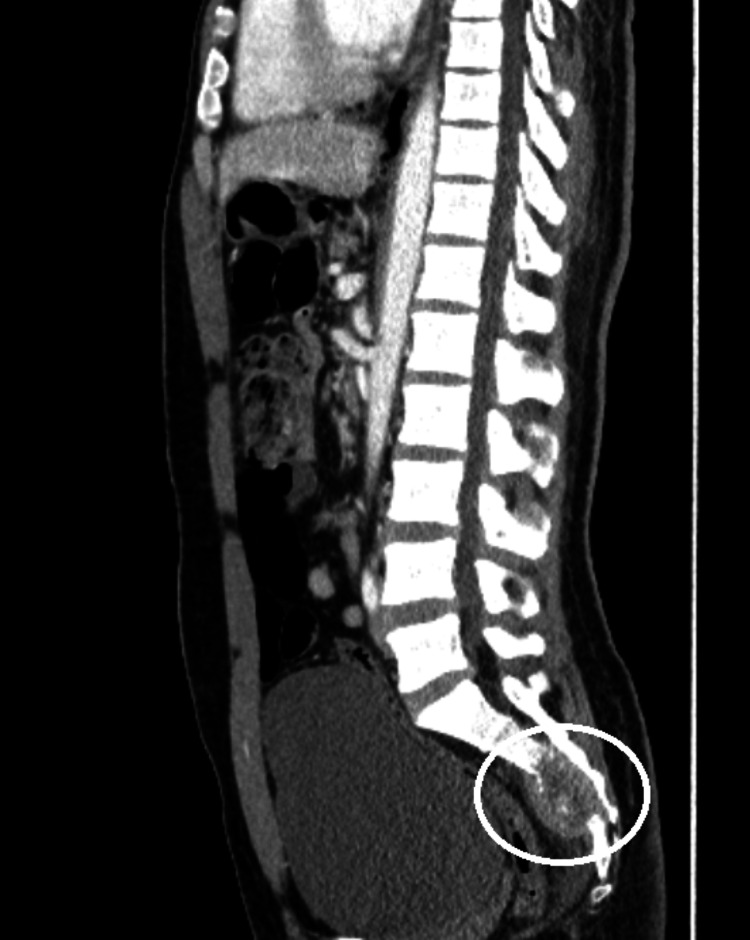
CT abdomen showing the sacrum with S3 and S4 demonstrating a lytic lesion CT: computed tomography.
